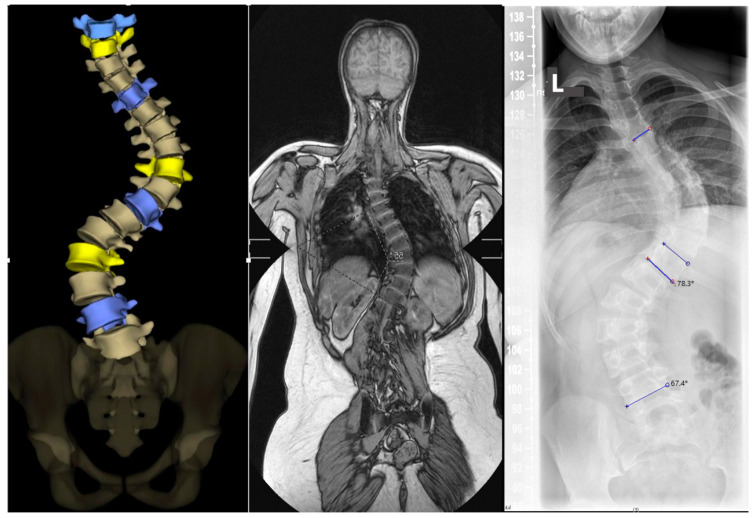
From left to right: EOS, MRI scout image, whole-spine X-ray. (Left image) Coronary EOS view, with color-coded apical vertebrae in yellow and neutral vertebrae in purple. (Middle image) Coronary MRI image in T1W sequence for planning the thin-layer 3D sequences. (Right image) X-ray standing image for determining the Cobb angle. The blue lines are reference lines for calculating the angles. The upper line is for the thoracic curve, the two middle lines for the major curve and the lower line for the lumbar curve.
